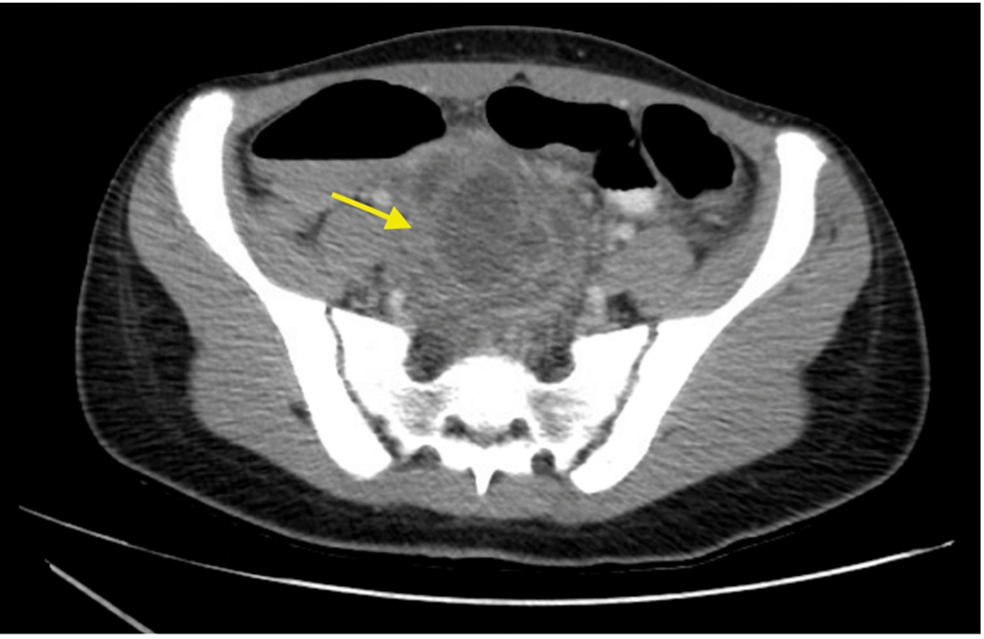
CT Axial view of the right lower quadrant pelvic mass
